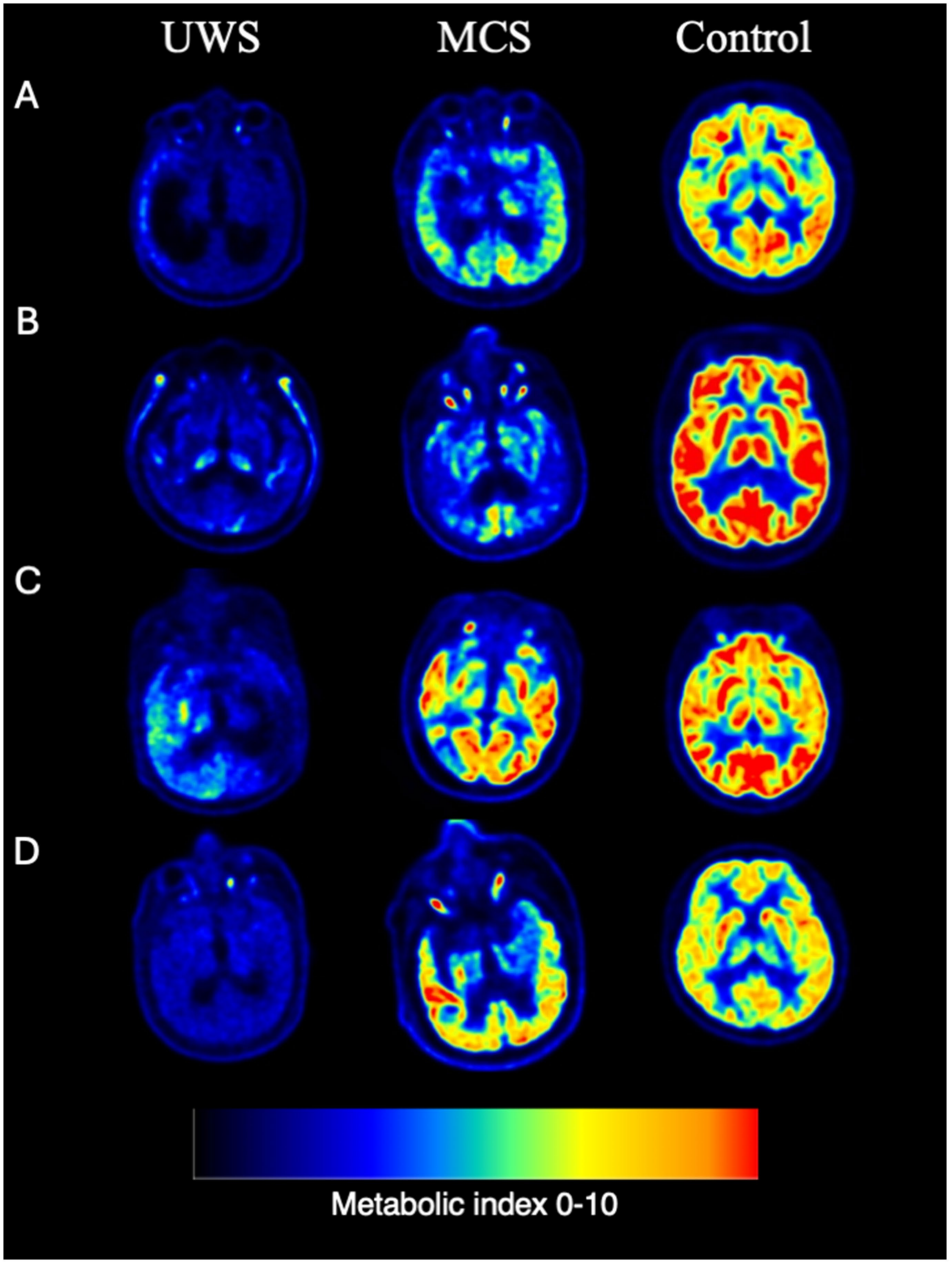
Representative individual FDG PET examples. (A) Cerebral trauma. (B) Ischemic hypoxic encephalopathy. (C) Cerebral hemorrhage. (D) Brain stem hemorrhage.
